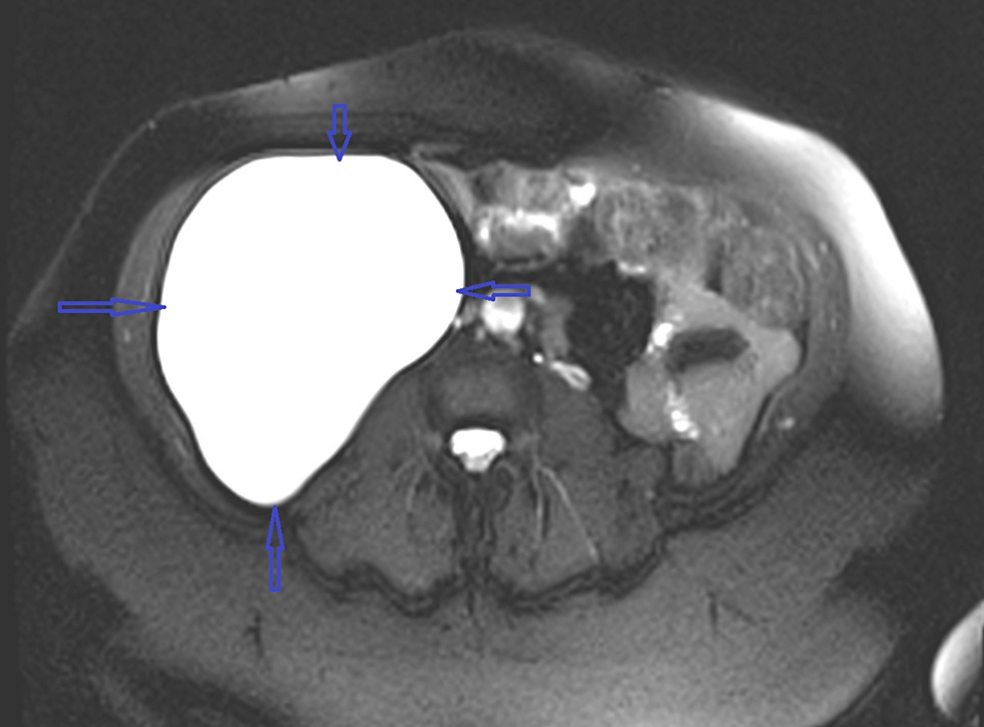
T-weighted MRI of abdomen image showing a 15x9x5 cm right retroperitoneal cystic mass MRI, magnetic resonance imaging
